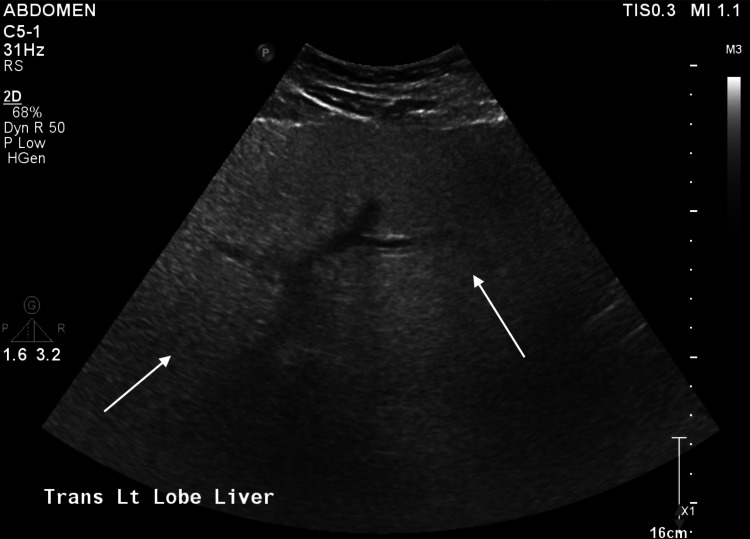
Liver ultrasound with hepatic steatosis
